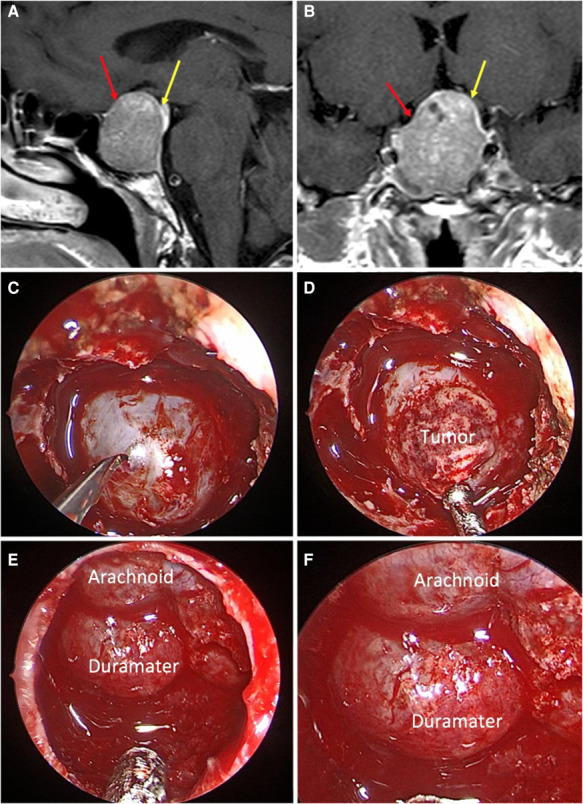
Mixed sellar barrier.* A 59-year-old female patient, with a PRL produced macroadenoma. (A,B) Preoperative MRI: the yellow arrows indicate the barrier that captures contrast with a thickness greater than 1 mm and the red arrows indicate the barrier that captures contrast with a thickness less than 1 mm. (C–F) Intraoperative images: the barrier constituted by duramater and arachnoid can be seen.
